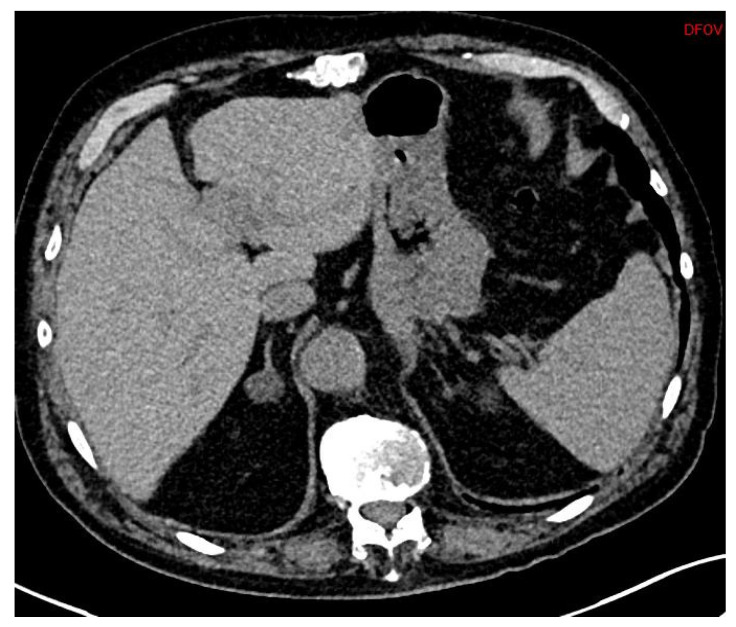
Abdominal CT (DFOV 80 × 35 mm): Adrenal adenoma, right adrenal nodule.
